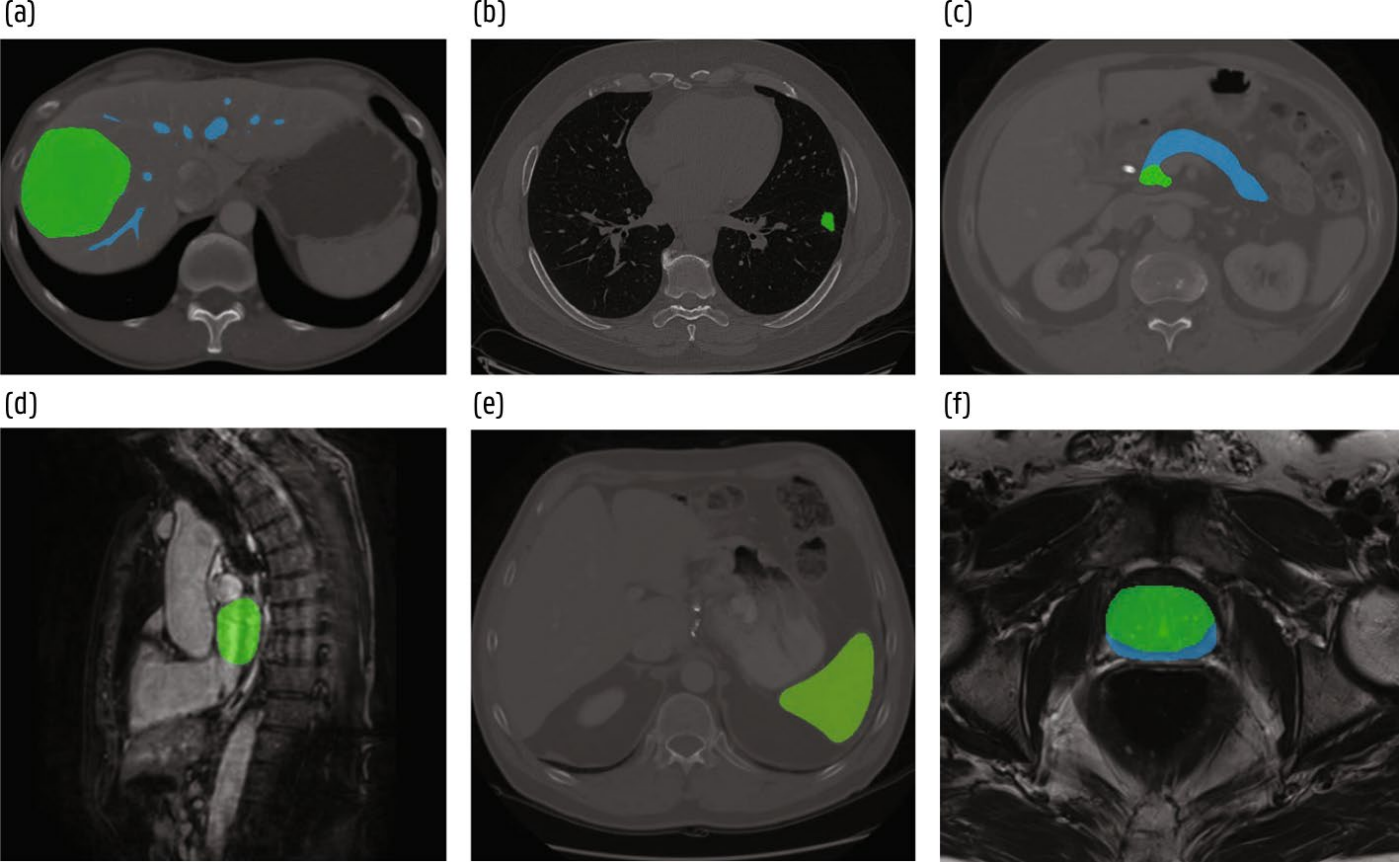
Segmentation examples from the Medical Segmentation Decathlon [134]. a Hepatic vessel (blue) and tumor (green) in CT. b Lung tumor (green) in CT. c Pancreas (blue) and tumor (green) in CT. d Left ventrical (green) in MRI. e Spleen (green) in CT. f Prostate peripheral (blue) and transitional (green) zones in MRI
